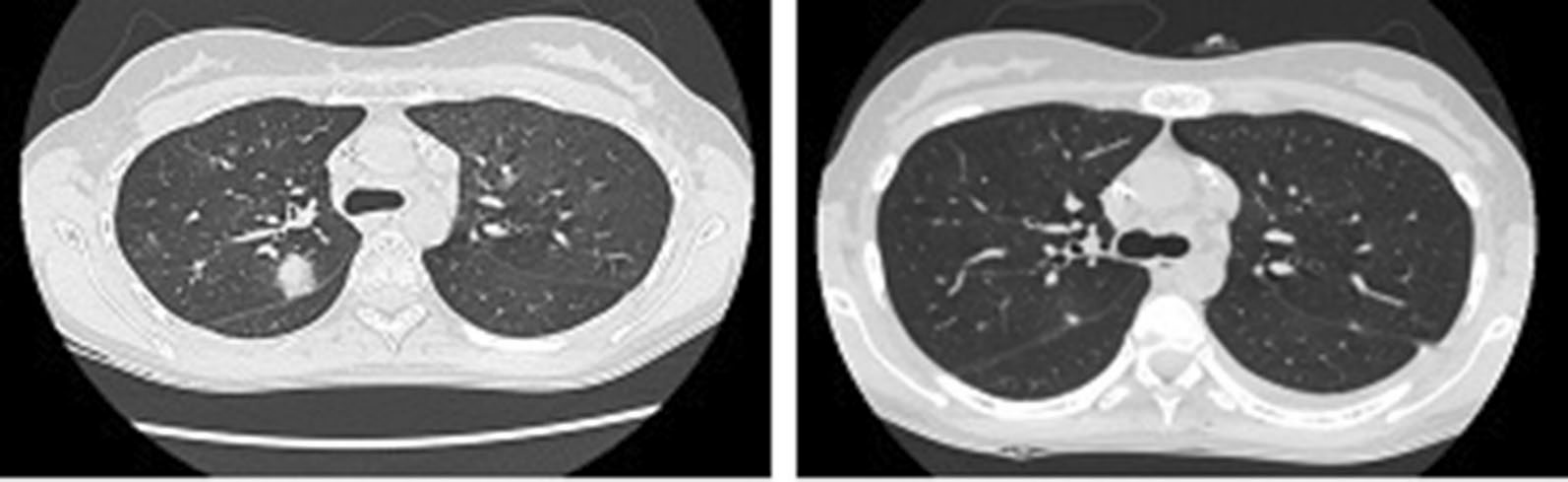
Left Pannel shows CT Scan at diagnosis of chronic lung GVHD. Right panel shows CT scan 3 months after ruxolitinb treatment
